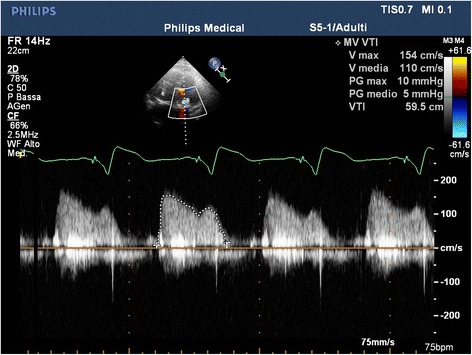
PreDischarge Trans-Thoracic echocardiography Mitral prosthesis’ gradient
